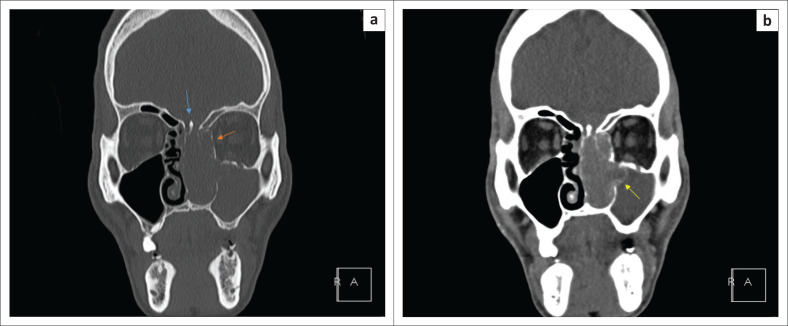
(a) Coronal CT bone window shows an expansile, polypoid mass in the left nasal cavity. Superiorly, the cribriform plate, olfactory recess and fovea ethmoidalis are eroded and widened (blue arrow). The nasal septum is deviated to the right. The left anterior ethmoid and frontal sinus air cells are opacified. There is convex bulging of the lamina papyracea, which is eroded (orange arrow). The infundibulum and middle meatus are eroded and widened. The left maxillary is opacified. The hard palate is eroded. (b) Coronal CT soft tissue contrast-enhanced image shows a uniformly contrast-enhancing polypoid tumour in the left nasal cavity, extending to the cribriform plate, olfactory recess and ethmoid sinus. No demonstrable intracranial extension. The left maxillary sinus outflow tract is widened and obstructed by a tumour extending into the infundibulum and middle meatus with a resultant effusion (yellow arrow).
